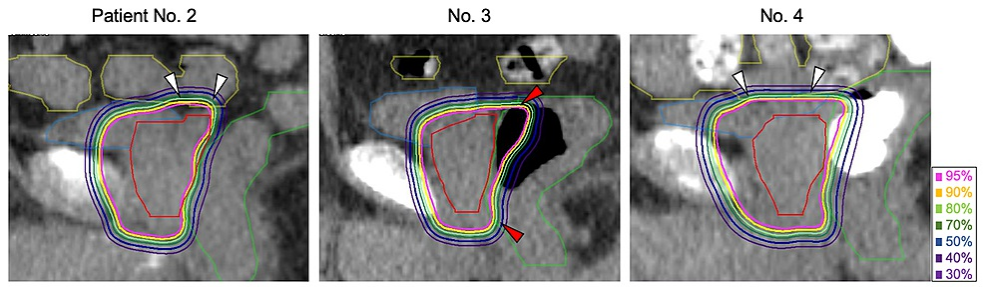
Dose distribution in cases wherein the dose constraint exceeded. In-room CT sagittal images of three patients are shown. Patients no. 2 and 4 received high doses to the nearby intestine (yellow line, white arrows). In patient no. 3, the rectum (green line) enlarged because the rectal gas was irradiated with a high dose (red arrows).
